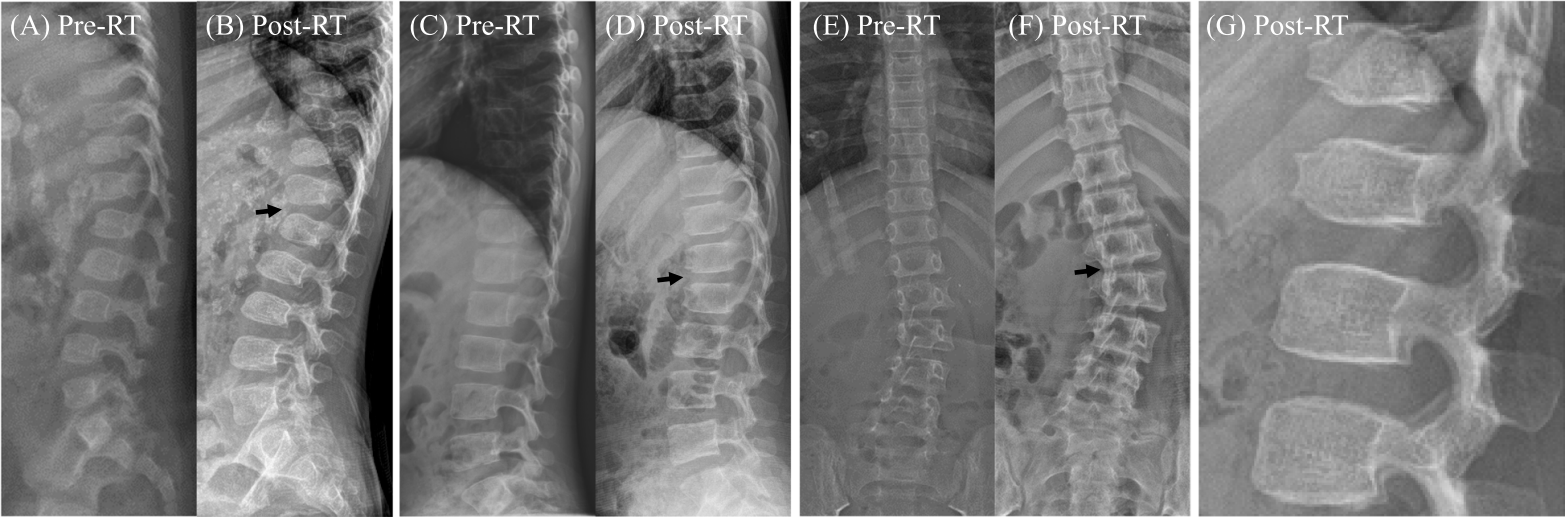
Pre-RT and post-RT (five years after RT) thoracolumbar X-ray films in four cases. The first child diagnosed with neuroblastoma at five months showed kyphotic features before RT (A), which was similarly observed post-RT X-ray (B). Mild kyphosis was newly observed on post-RT film (C and D) for the second child treated with RT at 87 months. The third case with RT at 106 months already showed scoliosis before RT (E), and it progressed at five years after RT (F). Irradiated upper vertebrae (T12-L1) were more irregular than unirradiated lower vertebrae (L2-L3) five years after RT in the youngest children who were diagnosed with neuroblastoma at birth and treated with RT at 13 months (G)
